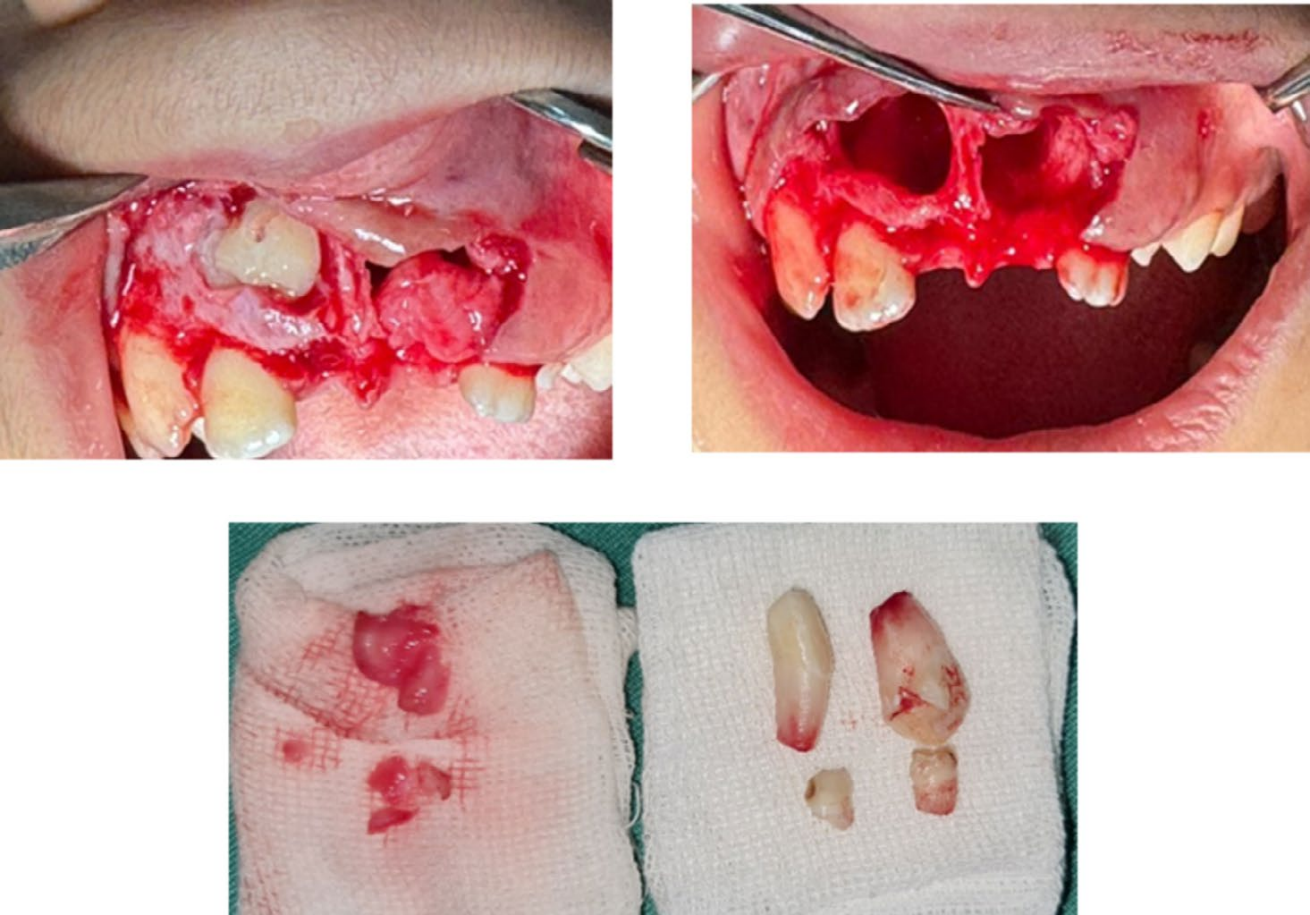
Intraoral clinical view of the exposed mesiodens following surgical flap elevation. The anomalous crown morphology confirmed the preoperative radiographic findings and guided extraction.
